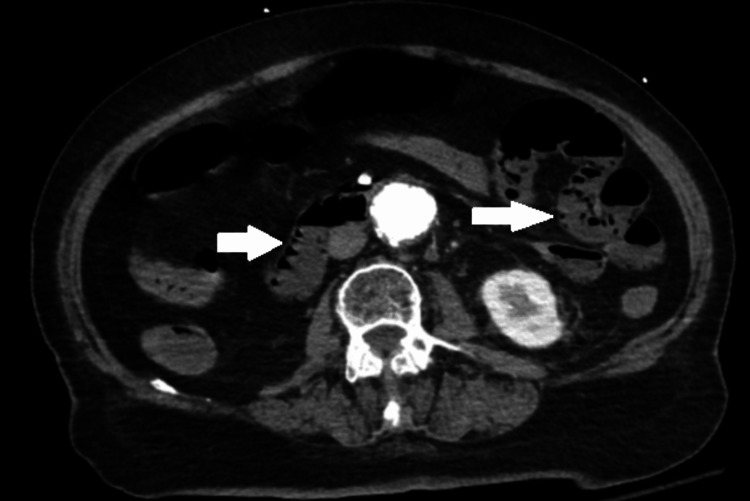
Computed Tomography (CT) of the Abdomen and Pelvis CT demonstrates acute duodenal and mesenteric pneumatosis (white arrows), suspicious for acute mesenteric ischemia with associated bowel necrosis.
